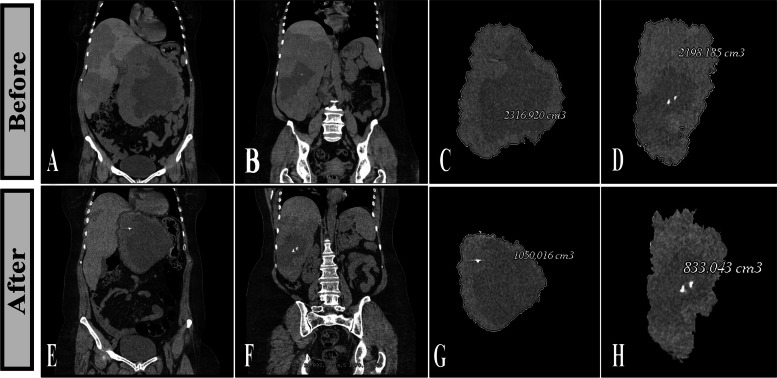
A, B A middle-aged woman with two giant HVMs in the left and right liver lobes with abdominal pain and early satiety. C, D Volumetry of the left and right lobe HVMs depicts HVM volume of 2316 mL and 2198 mL before the procedure, respectively. E, F Follow-up CT nine months after sclerotherapy shows size reduction in both lesions. G, H Post-treatment volumetry shows more than 50% volume loss in both lesions, with a volume of 1050 and 833 mL in the left and right lesions, respectively. The patient reported significant relief of upper abdominal pain
